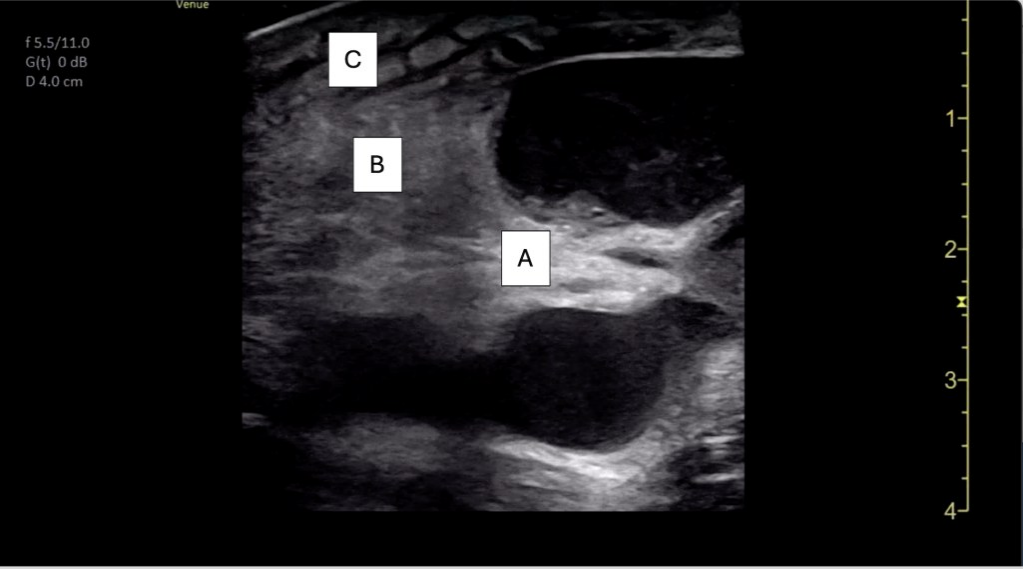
Point of care ultrasound (POCUS) image demonstrating posterior acoustic enhancement (A), soft tissue edema (B), and superficial cobblestoning (C).
